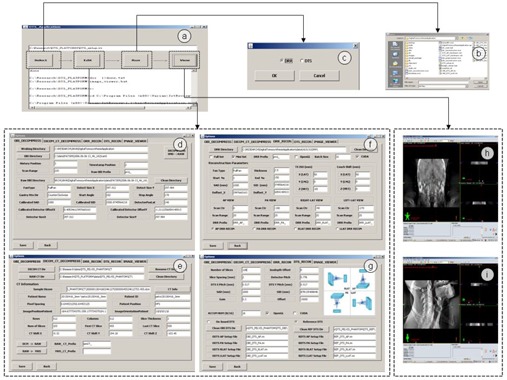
The user interface of DTS software tool: (a) main window; (b) window of file selection; (c) window of DRR and DTS reconstructions selection; (d) window of parameter configuration for cone‐beam projection decompression; (e) window of parameter configuration for planning CT decompression; (f) window of parameter configuration for DRR reconstruction; (g) window of parameter configuration for DTS reconstruction; (h) demonstration of image registration between coronal ODTS and coronal RDTS; (h) demonstration of image registration between sagittal ODTS and sagittal RDTS.
